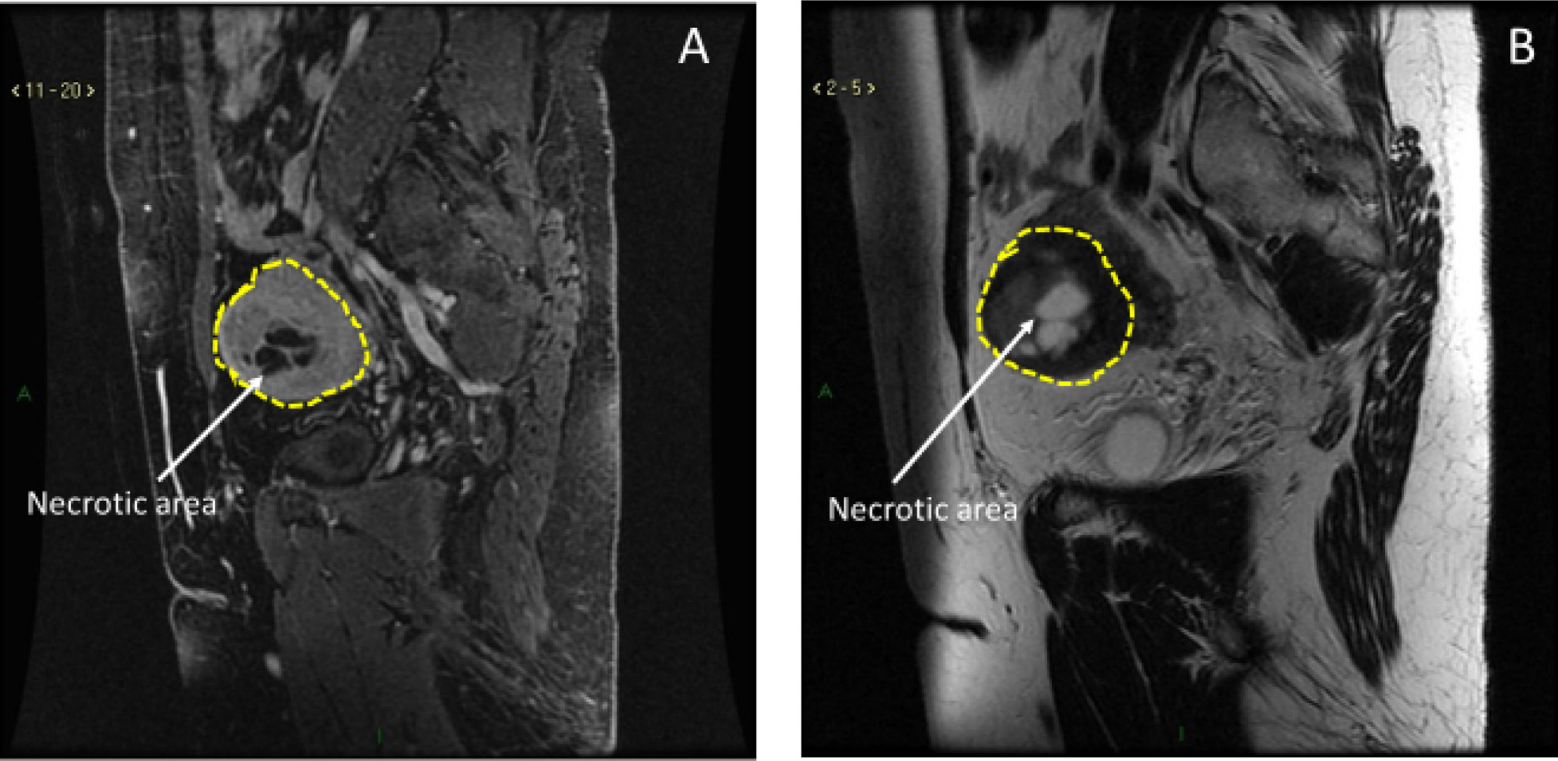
MRI characteristics of uLMS (A) Hyperintense signal in T1-weighed images. (B) Hyperintense signal in T2-weighed images.
